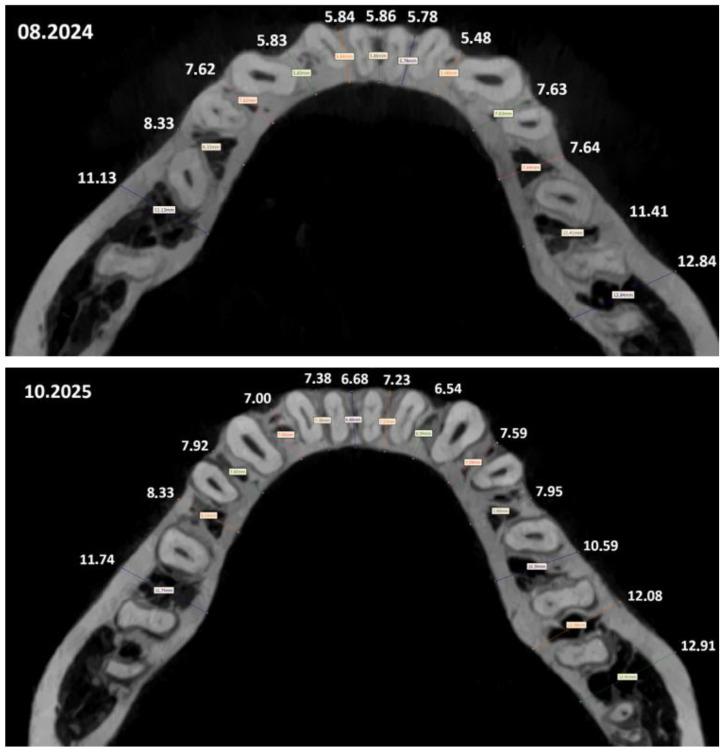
Mandibulae alveolar ridge width before and after treatment (CBCT).
